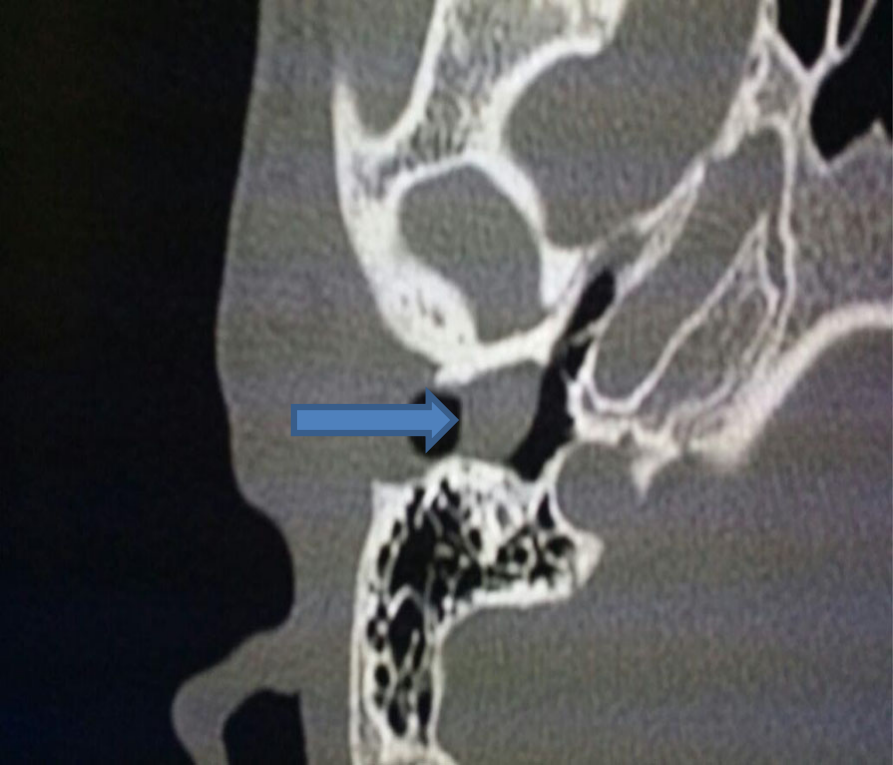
A computed tomography scan of the temporal bone showing a well-circumscribed, soft tissue mass narrowing most of the external auditory canal (arrow) with no bone erosion and no middle ear or mastoid involvement
